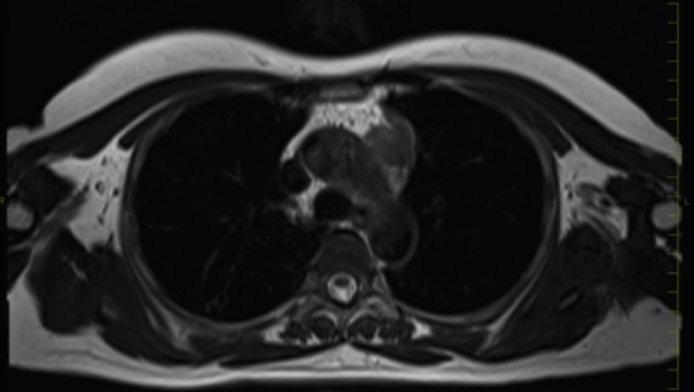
Magnetic resonance imaging of the chest shows high intensity on T2-weighted images with suspected invasion to the upper lobe of the left lung.
